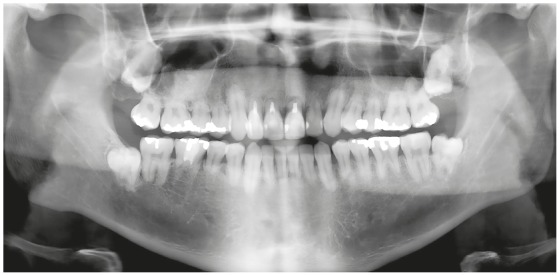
Endodontic treatment of three teeth that had extreme inflammatory root resorption after four years of orthodontic treatment was unnecessary, as shown in digital panoramic radiograph. Same clinical case as in Figure 8, at seven years of follow-up. Source: Consolaro and Furquim^3^, 2014.
